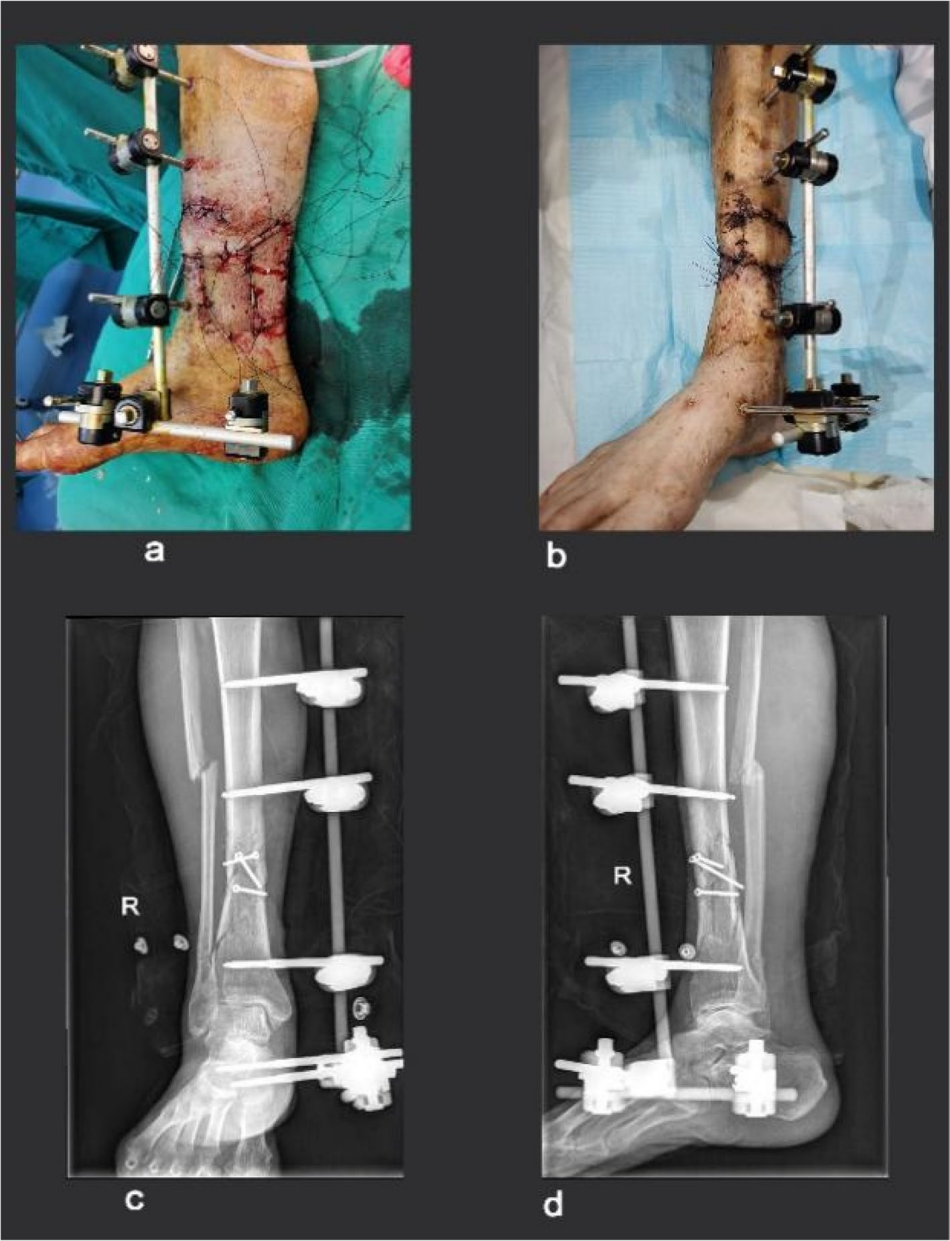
75 years old male with an open fracture of the middle and lower segment of the right tibia and fibula. a Day of operation, (b) 6.^th^-day post-operation, (c) and (d) Are Computed tomography at post-operation day 7
